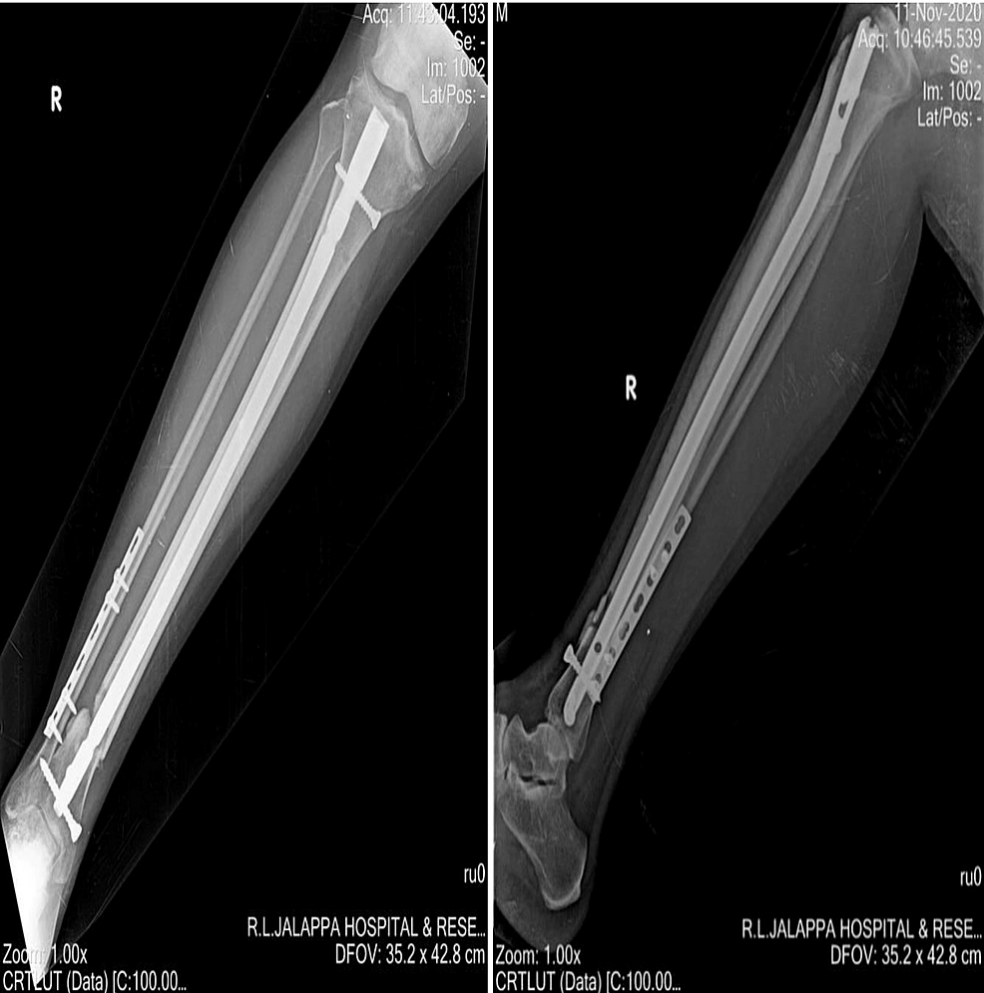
Nine months follow-up AP and lateral radiographs of a 36-year-old smoker showing nonunion of the distal third fracture of the right tibia with IMIL nail in situ
